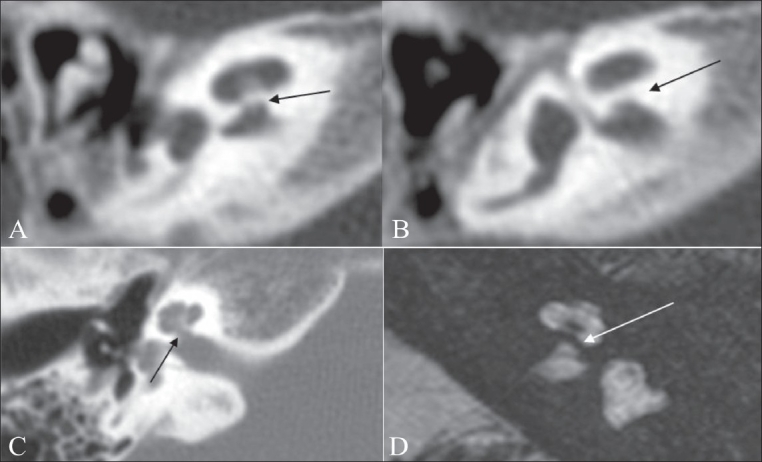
Isolated cochlea. Axial HRCT images (A, B) show a thick bony bar at the cochlear aperture (arrow), suggesting an isolated cochlea. An axial HRCT image (C) shows a normal appearance of the cochlear aperture at the site of the entry of the cochlear nerve, near the modiolus. An axial 3D-FIESTA image (D) shows a bony bar (arrow) at the cochlear aperture (isolated cochlea). Also note the left cochlear nerve deficiency
